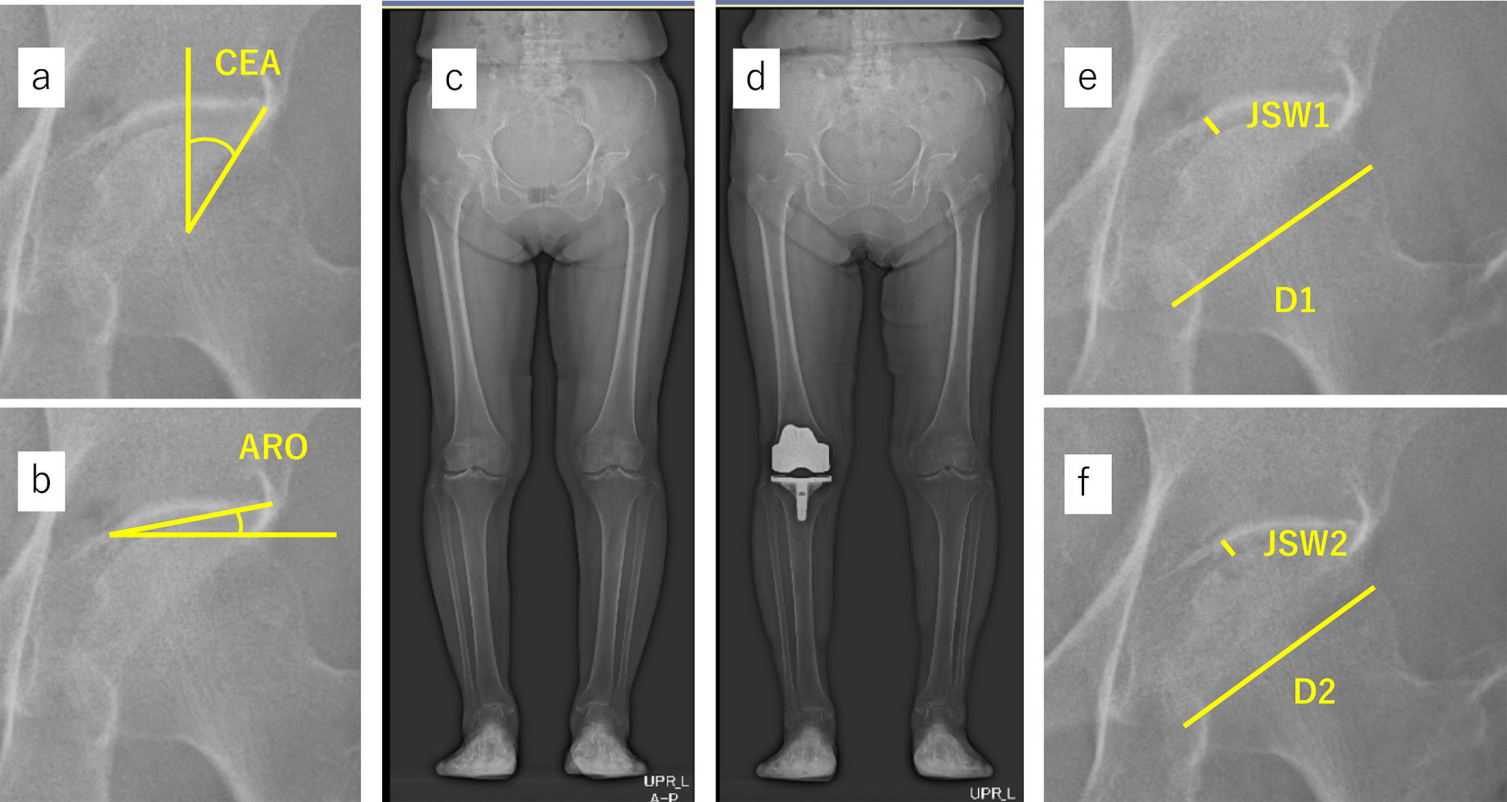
Measurements of the a) centre-edge angle (CEA), b) acetabular roof obliquity (ARO), and hip joint space width (JSW) in a representative case (75-year-old female). c) Anteroposterior (AP) standing whole-leg radiograph taken before surgery. d) AP standing whole-leg radiograph taken five years after surgery. e) Magnified image of the area including the right hip in panel c). f) Magnified image of the area including the right hip in panel d). The JSW and the femoral head diameter were measured as indicated by the yellow lines. JSW1 and JSW2 are the JSWs before surgery and at the final follow-up, respectively. D1 and D2 are the femoral head diameters measured on radiographs at three months after surgery and at the final follow-up, respectively.
